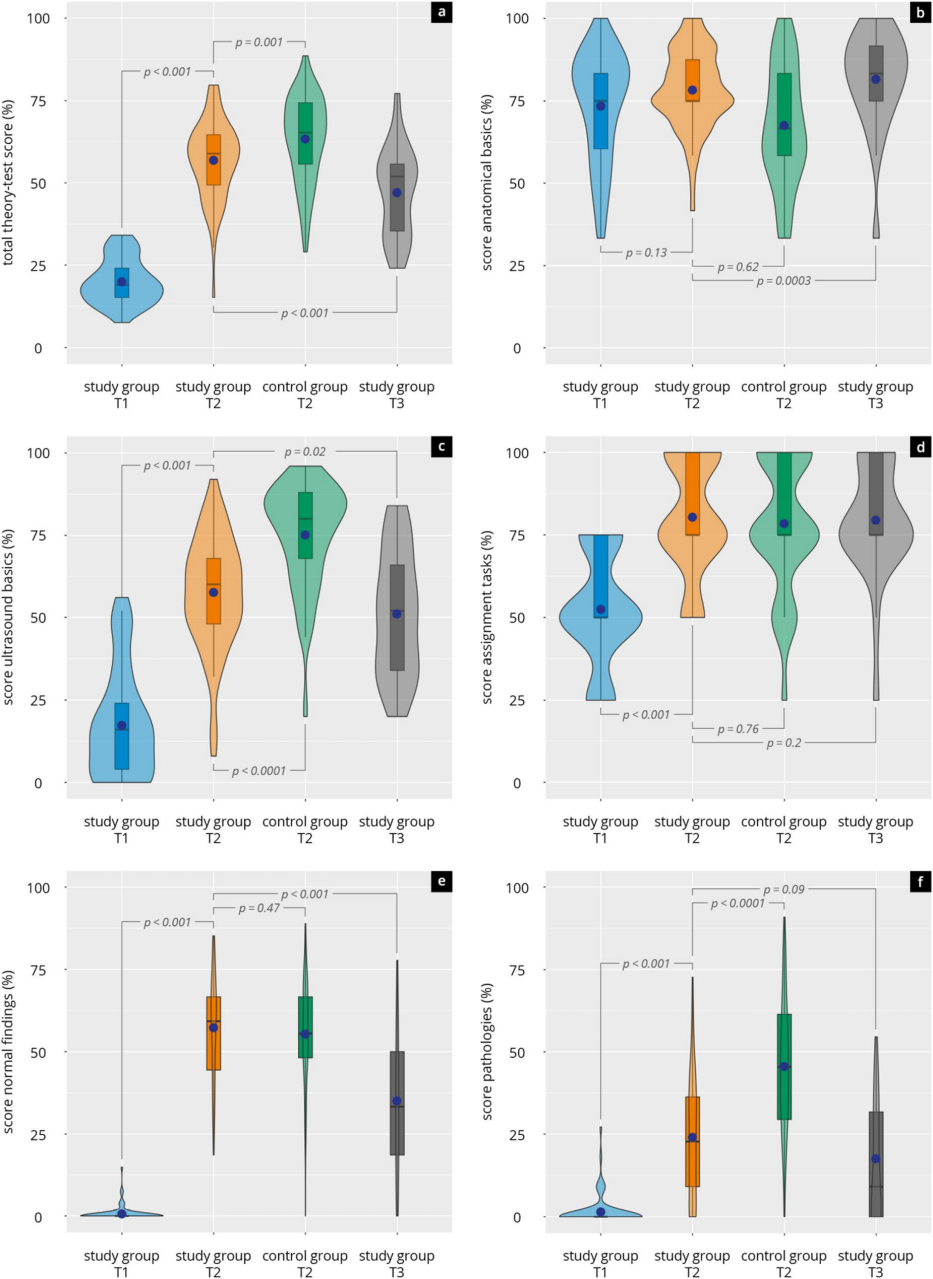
Results of the theory test in the study group (students) and the control group (physicians) at time points T1, T2, and T3. Shown are violin plots (with median [black bar], interquartile range, and mean [blue dot]) for (a) total score, b anatomical basics, c ultrasound basics, d assignment tasks, e normal findings, and (f) pathologies. Significance levels of group comparisons are indicated
